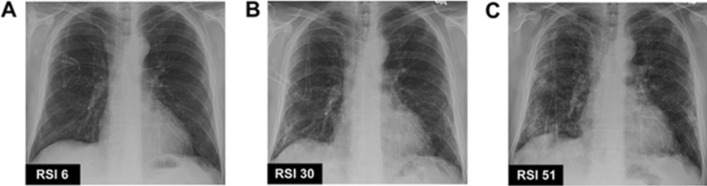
Representative radiologic severity index score serial for chest X-ray. RSI scores are labelled within each panel. Panels A–C show chest X-ray images from an individual patient with parainfluenza virus-associated lower respiratory infections from the previous study in America, in order of increasing severity [4]
